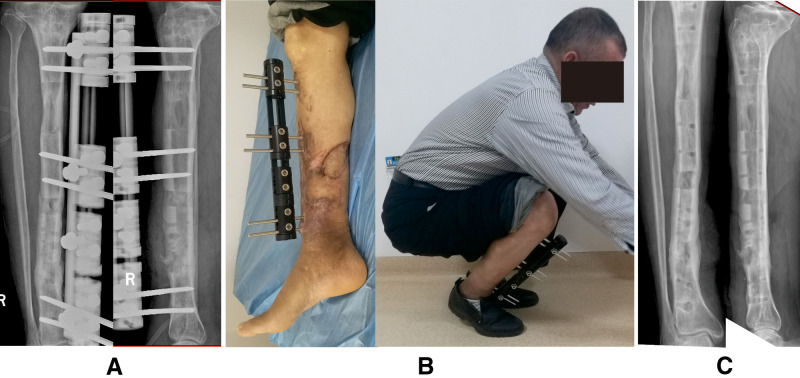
Images of the same patient shown in Figure 4. (A) Complete consolidation and docking site union after docking in 3 months. (B) Satisfactory functional recovery before monolateral external fixator removal. (C) Radiographs 9 months later after removing the frame.
